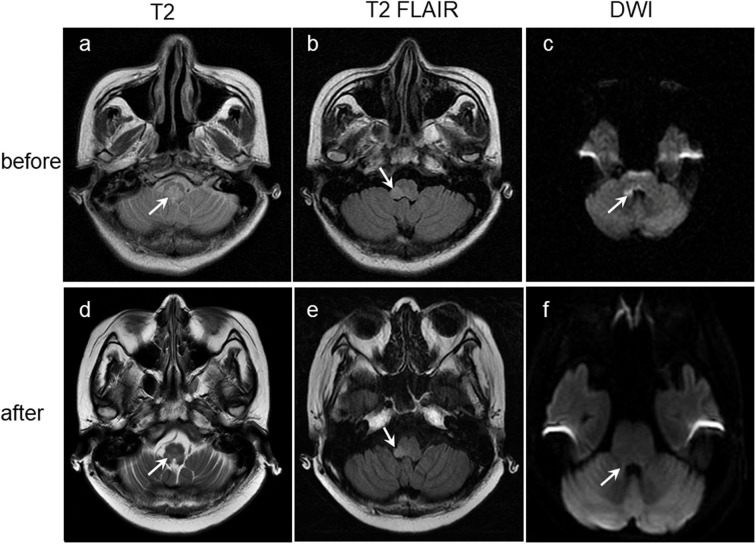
Lesions (arrowed) on brain coronary MRI images before (a–c) and after (d–f) antiviral treatment showing obviously manifested shrinkage after antiviral treatment. T2-weighted: Lesions on the medulla oblongata (a,d). T2-FLAIR-weighted: Lesions on the right arm of medulla oblongata (b,e). DWI: Lesions on right side of the right corpora quadrigemina, right side wall of the fourth ventricle and right side of the medulla oblongata (c,f). DWI, Diffusion-weighted MRI.
